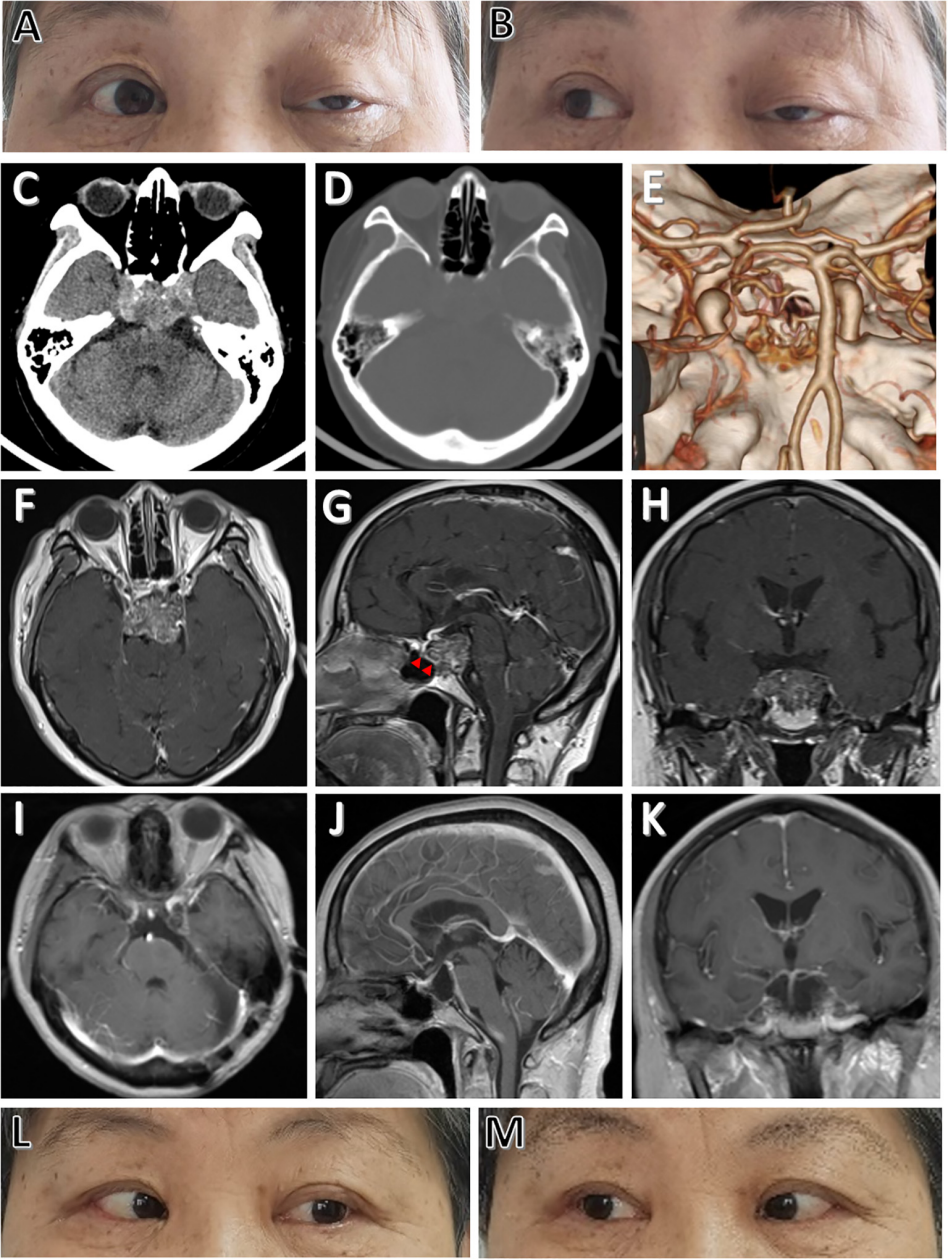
Physical and radiological evaluations of the patient preoperatively and postoperatively. (A, B) Preoperative physical examinations of the patient showing cranial nerve paralysis of left CN III and CN VI. (C) Preoperative CT image showing a hyperdense lesion located at the upper clivus. (D, E) Preoperative CT and CT angiography images showing clival bone destruction. (F-H) Preoperative post-contrast (gadolinium-enhanced) axial (F), sagittal (G), and coronal (H) MRI images showing thick enhancement of the lesion. (I-K) Postoperative post-contrast axial (I), sagittal (J), and coronal (K) MRI scans performed one year after surgery showing total removal of the lesion. (L, M) Postoperative physical examinations showing complete functional recovery of CN III and CN VI paralysis at one-year follow-up. The red arrowheads indicate the intact thin layer of membrane structure separating the tumor from the sphenoid cavity.
